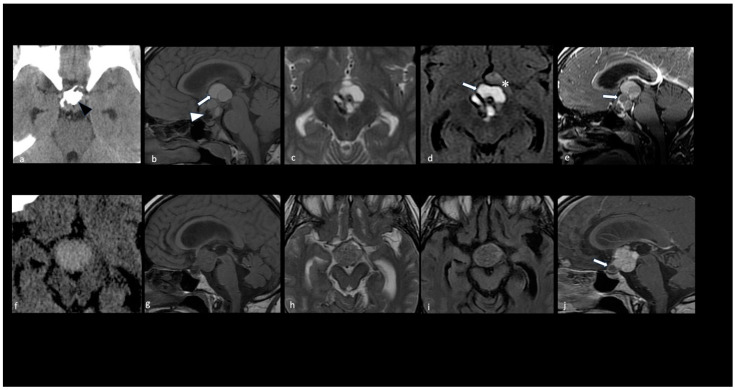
Key morphologic features in adamantinomatous and papillary craniopharyngiomas. CT scan (a,f); sagittal T1 (b,g), axial T2 (c,h), axial FLAIR (d,i), and sagittal T1 with contrast medium (e,j) MRI. Adamantinomatous craniopharyngioma (a–e). Note a predominantly cystic multilobulated suprasellar mass with an intratumoral coarse calcification pattern (black arrowhead in (a)). The MRI shows the following: T1 shortening and FLAIR/T2 hyperintensity within the multicystic tumor component due to machine oil-like proteinaceous fluid (white arrows in (b,d)); T1 hypointense elements representing calcifications (white arrowhead in (b)); rim enhancement of the wall of the cysts on T1 with a contrast medium (white arrow in (e)). Parenchymal perifocal edema-like changes along the left optic tract and chiasm are shown in (d) (*). Papillary craniopharyngioma (f–j). Note a predominantly solid suprasellar mass without evidence of calcifications. The solid component appears hyperdense on the CT (f) with a homogeneous enhancement; the small cystic component is caudal and shows the rim enhancement of the wall (white arrow in (j)).
